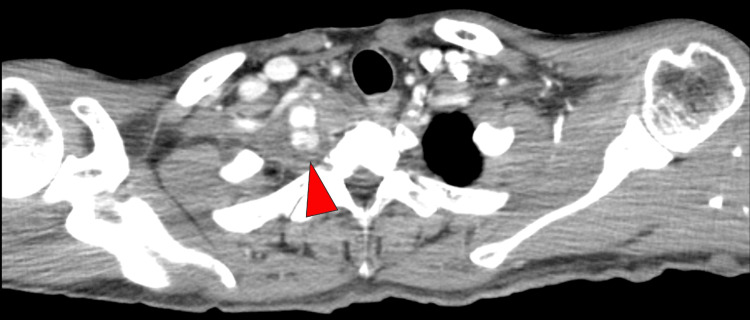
Hemothorax and contrast leakage Contrast-enhanced computed tomography scan. The right hemothorax and contrast leakage near the right thyrocervical trunk (arrowhead)
